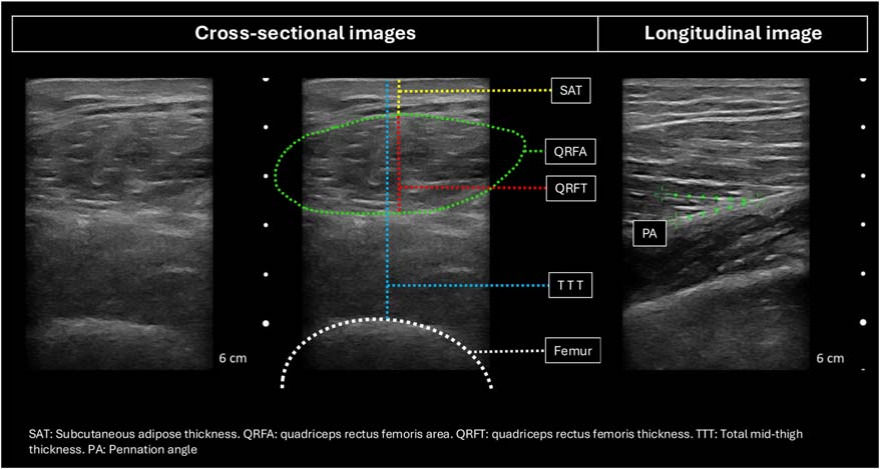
Ultrasound geometric muscle measurements.
